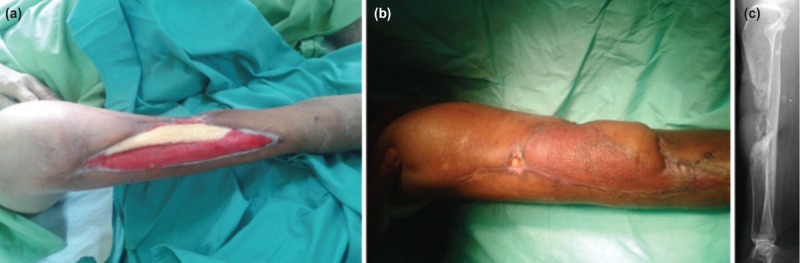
(a) A 58-year old patient with diabetes with exposed and dried shaft of tibia following debridement for necrotising soft tissue infection. (b) Patient developed persistent discharging sinus following GMCF surgery but refused further surgical intervention. (c) Plain radiograph a year after surgery showing osteolytic lesion at the midshaft of tibia secondary to chronic osteomyelitis.
